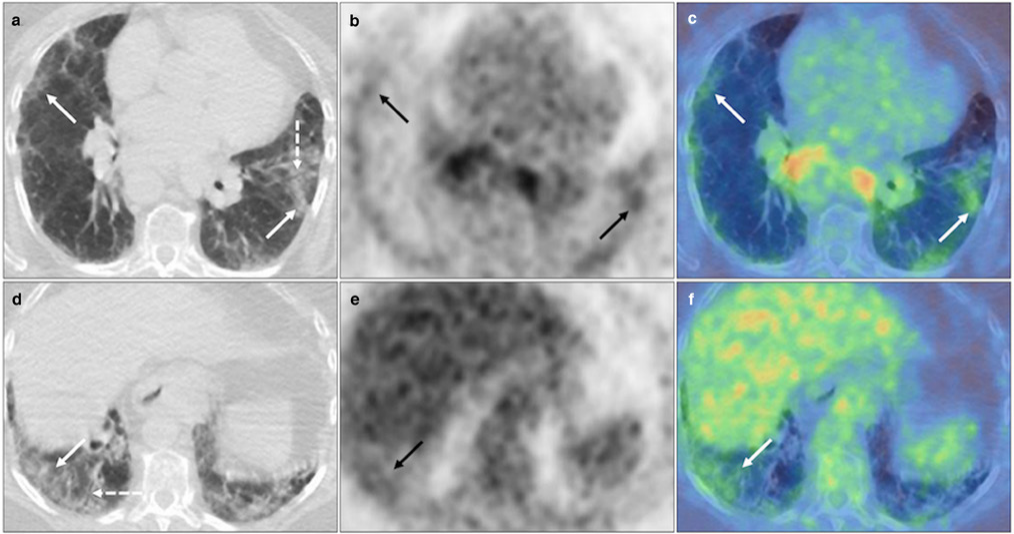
Bilateral FDG avid subpleural GGO with lower zone predominance (solid black and white arrows), *e.g.,* left lower lobe (SUV_max_ 3.4) and subtle co-existent tractional airways dilatation (dashed white arrows) on axial CT, PET and fused PET-CT (A-C, 5 F). BSTI 2 but appearances represented pulmonary fibrosis secondary to sarcoidosis in a *control* case.
